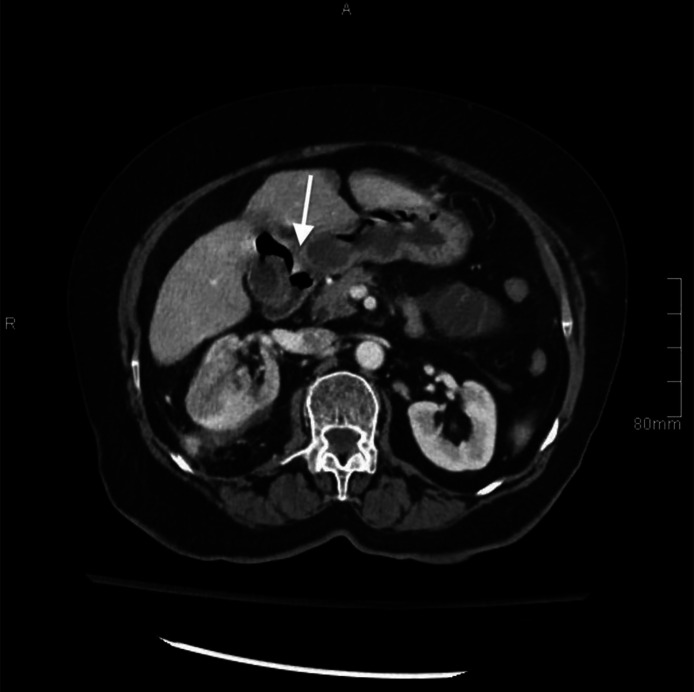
Axial contrast-enhanced CT image. The image shows a partially distended gallbladder with internal air foci representing fistulization of the first part of the duodenum (white arrow).
